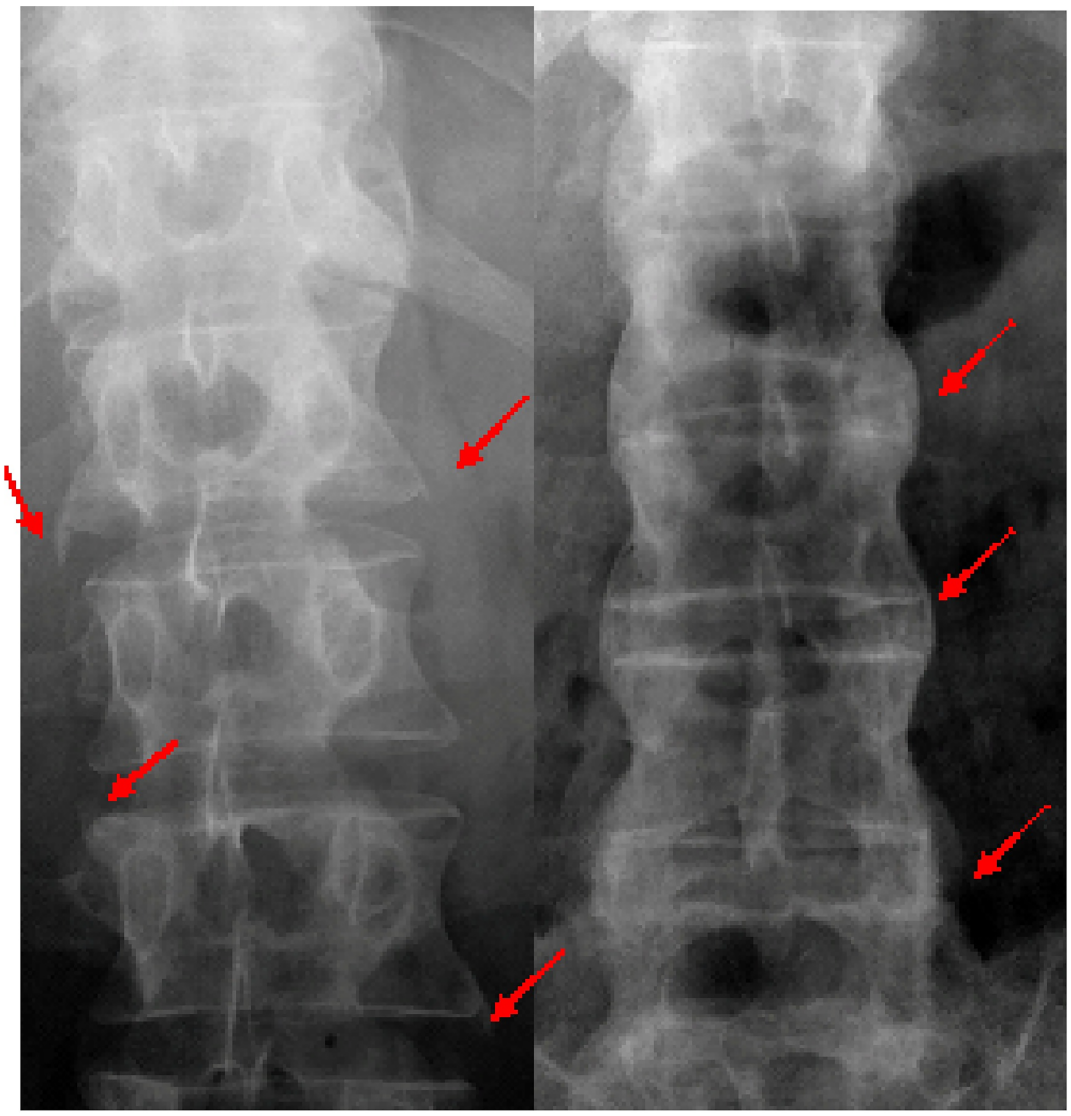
Syndesmophytes in the spine. On the left: Growing syndesmophytes in the spine of a 30-year old male with ankylosing spondylitis. On the right: Multiple syndesmophytes, bridging adjacent vertebral bodies in the spine of a 37-year male with ankylosing spondylitis, responsible for the so called bamboo spine image on X-ray.
